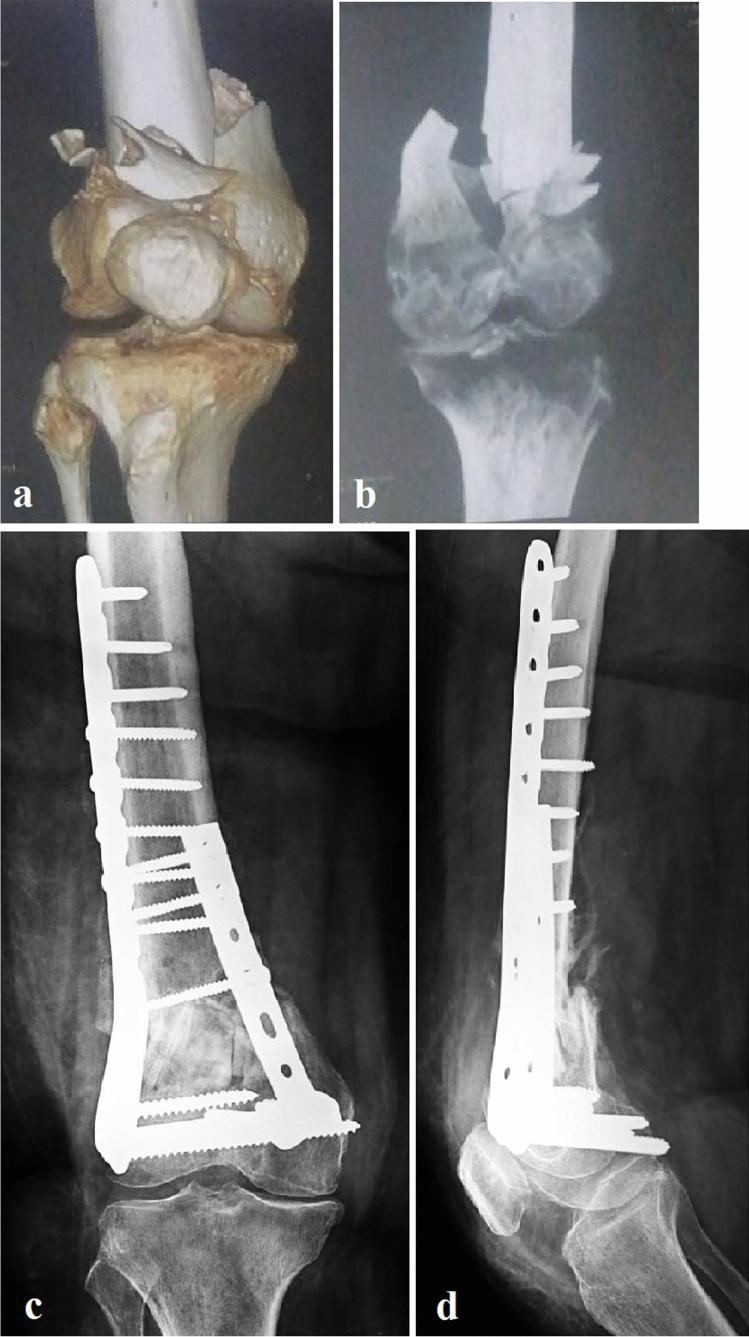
Male patient 75 years old, Sanders functional scoring 37 points with ROM 120°, no pain, and unrestricted walking at the end of the follow-up period (29 months). a Preoperative 3D CT scan showing a 33-C2 AO/OTA distal femur fracture. b Preoperative CT scan showing posterior comminution with intercondylar extension. c Last follow-up AP PXR showing anatomically reduced and fully united fracture. d Last follow-up Lateral PXR showing fully fused fibular graft
